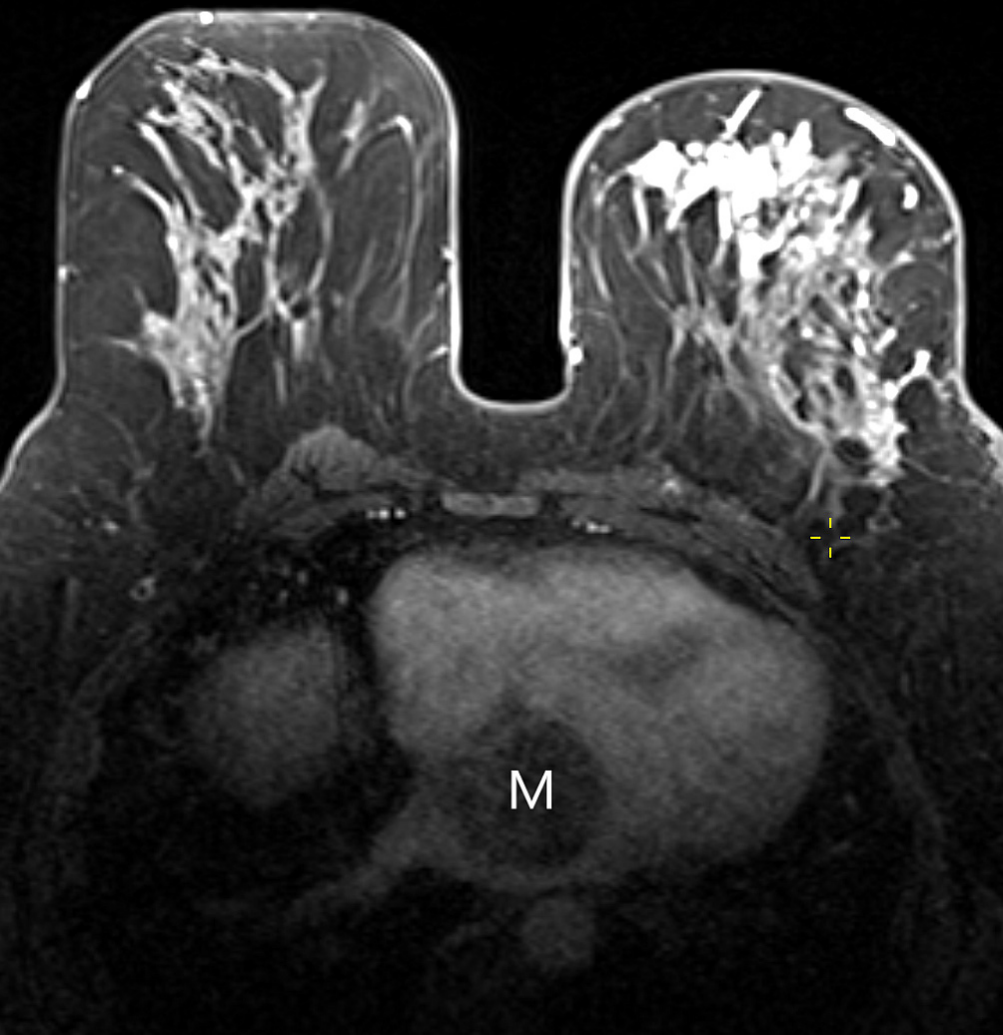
Incidentally detected left atrial myxoma (M) on breast MRI in a
